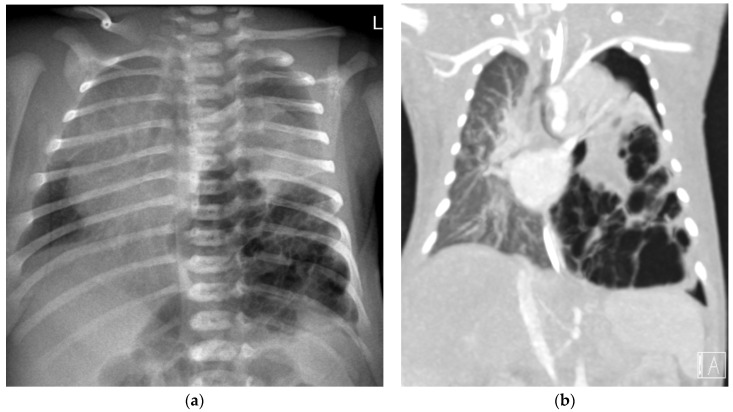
Two-day-old infant with respiratory distress. (a) Chest X-ray and (b) Coronal image of CTA: Large multilocular cystic lesion in the right lower hemithorax, with mediastinal shift to the right. There is an atelectasis of the left upper lung and apical pneumothorax.
